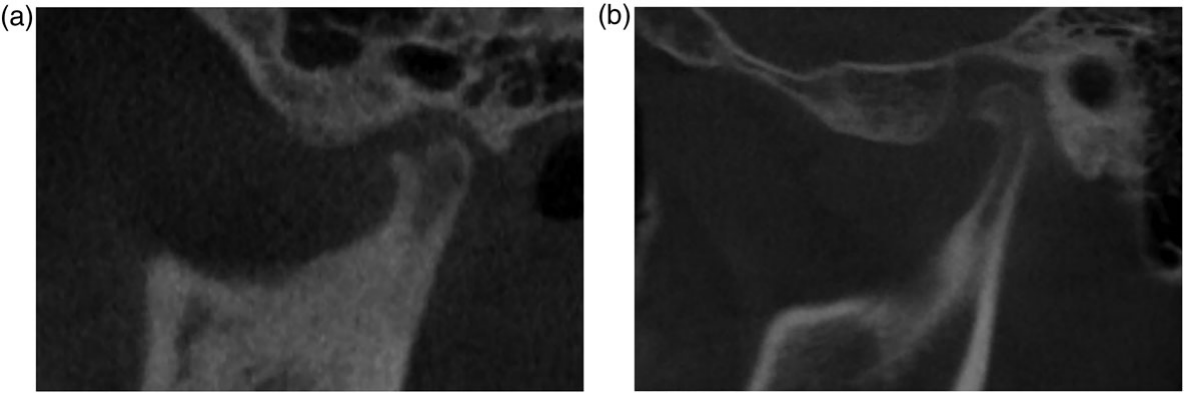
Representative sagittal cone beam computed tomography (CBCT) images showing temporomandibular joint osteoarthritis status. (a) A representative sagittal CBCT image of a 14‐year‐old female with condylar surface irregularity and erosion. (b) A sagittal CBCT image of a 15‐year‐old male with a typical osteophyte formation
